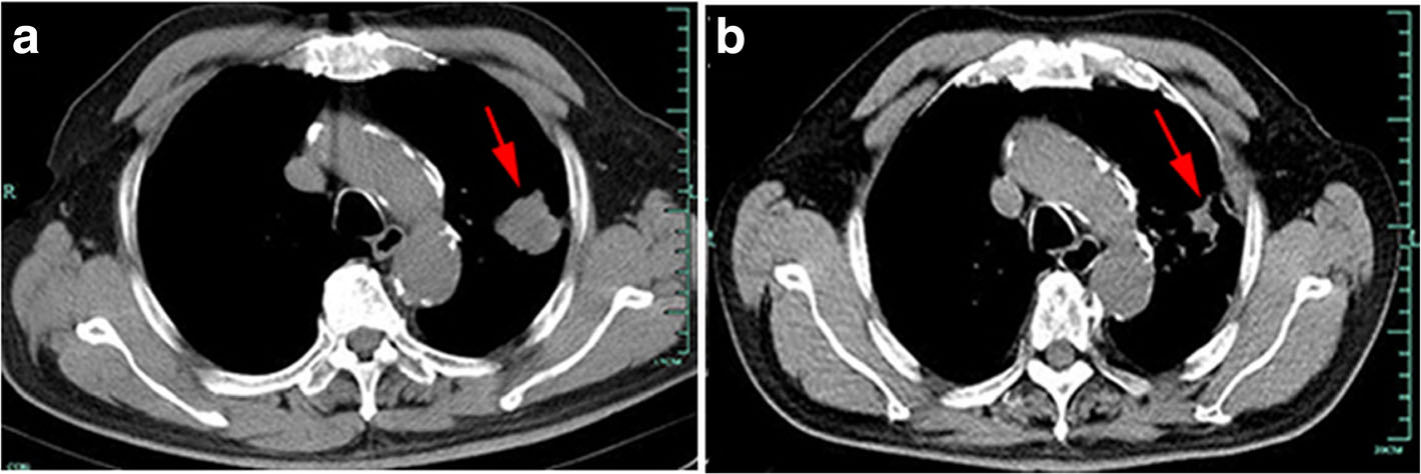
a Chest computed tomography scan showed a mass in the upper lobe of the left lung (*red arrow*). b A chest computed tomography scan revealed that there was a reduction in the size of the mass in the upper lobe of the left lung after radiotherapy (*red arrow*)
